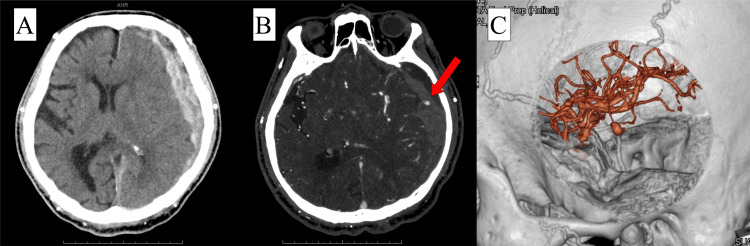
Preoperative images A: Computed tomography (CT) scan of the brain showing a 15-mm acute subdural hematoma on the left side. B and C: CT angiography with contrast showing contrast extravasation, raising concern for aneurysmal rupture (red arrow).
